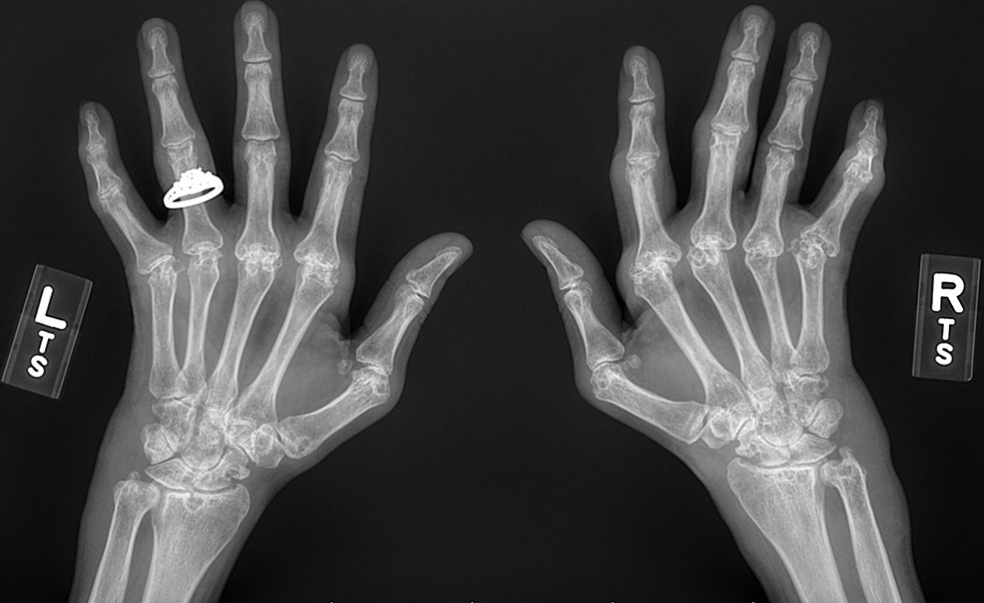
Bilateral hand x-ray demonstrating erosive arthritis, most notable in the metacarpophalangeal joints and wrists, along with ulnar deviation of the digits.
